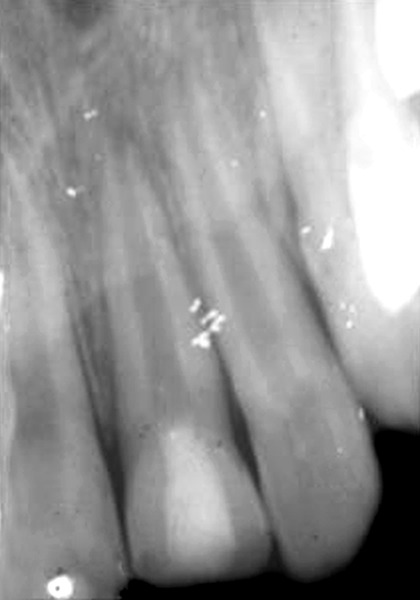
Radiograph immediately after pulpotomy
